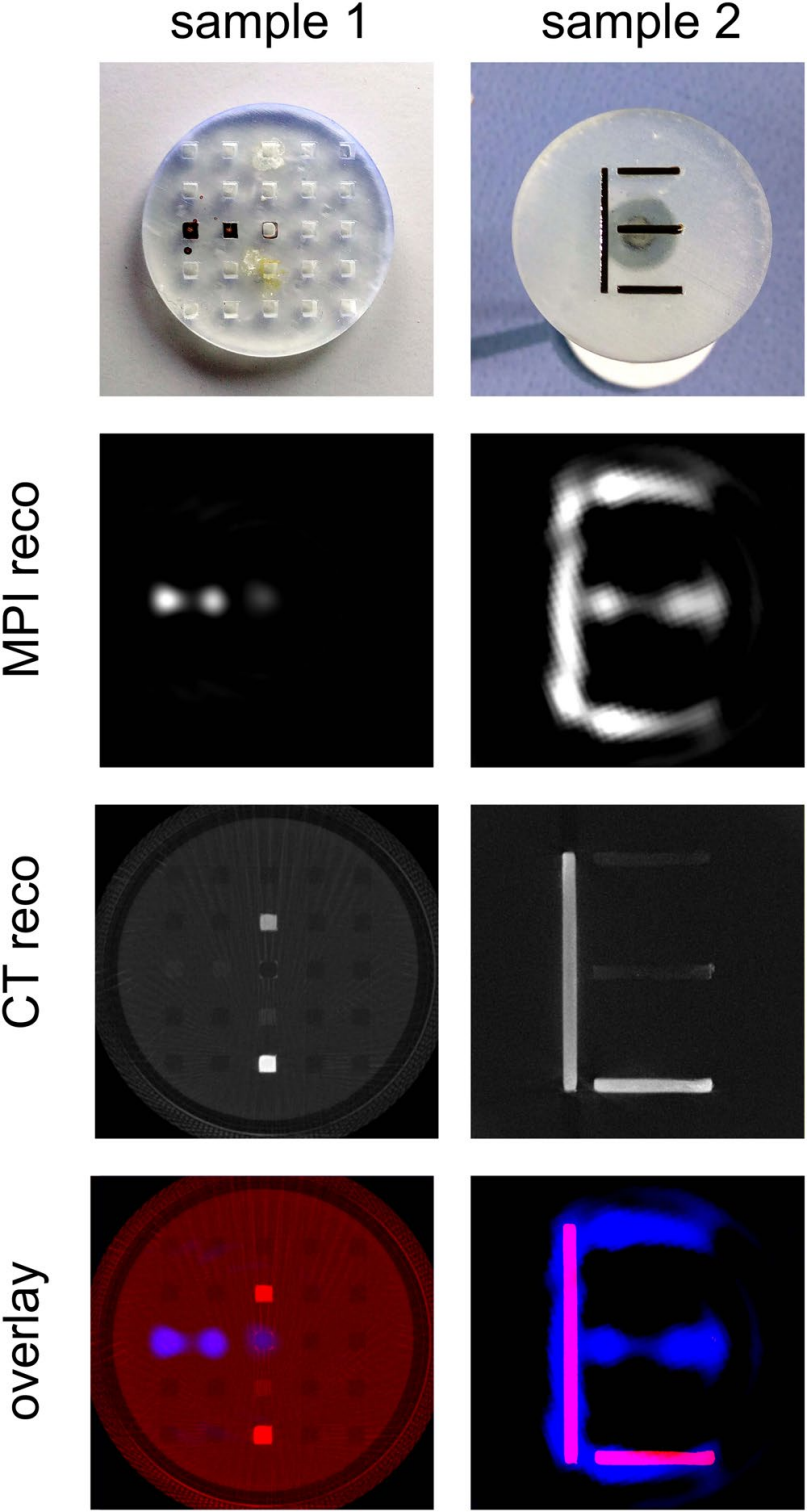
Results of simultaneous MPI CT measurement of sample 1 (left) and sample 2 (right).
